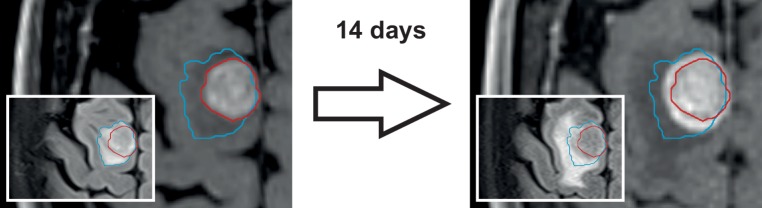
Importance of the time interval between MR imaging and treatment delivery. Example of growth in a melanoma brain metastasis that would have resulted in geographic miss. The 1.5 cm-diameter tumor increased from 2.1 to 3.0 cm^3^ during a time interval of 14 days (postcontrast T1-MPRAGE). *Note also:* Substantial increase in perifocal edema (inset: T2-FLAIR)
